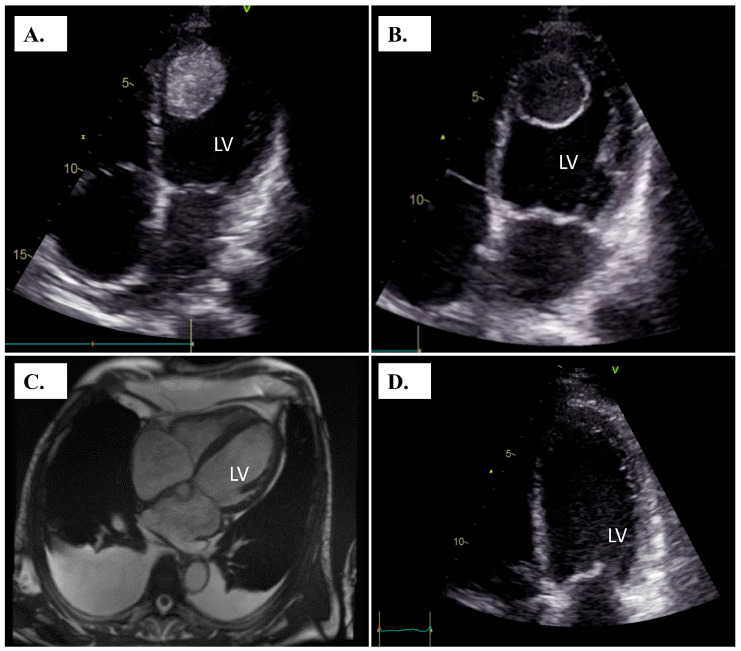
Echocardiogram at the time of hospitalization reveals a large mass in the apex of the left ventricle (A). Three days later, the morphology of the mass had changed with a denser core surrounded by a shell with a brighter signal (B). One week later, the cardiac MRI showed no evidence of left ventricular thrombus (C). No thrombi were seen later during the hospitalization or during the first outpatient visit (D). LV = left ventricle.
